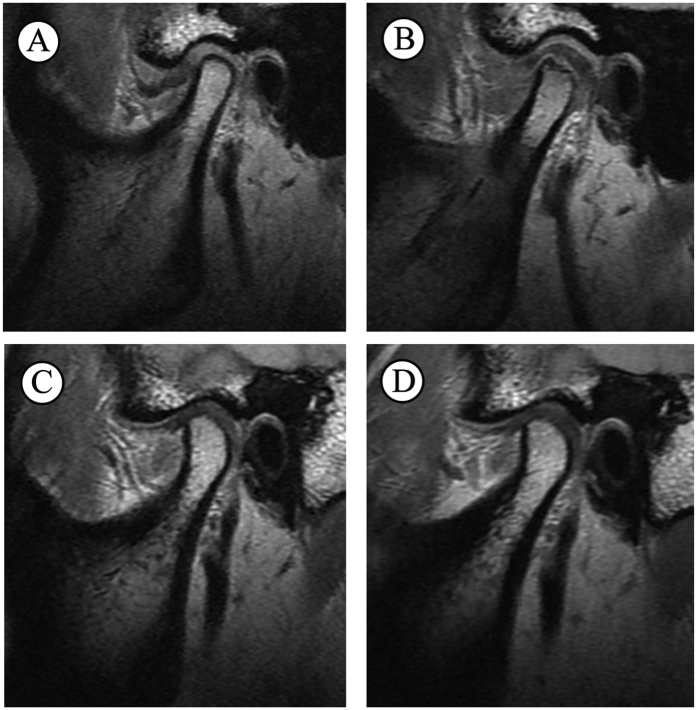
MRI scans of a 42-year-old female patient with ADD on the left side, with the follow-up period of 4 months. (A) Operative side preoperatively, (B) Operative side postoperatively, (C) Healthy side preoperatively, (D) Healthy side postoperatively.
